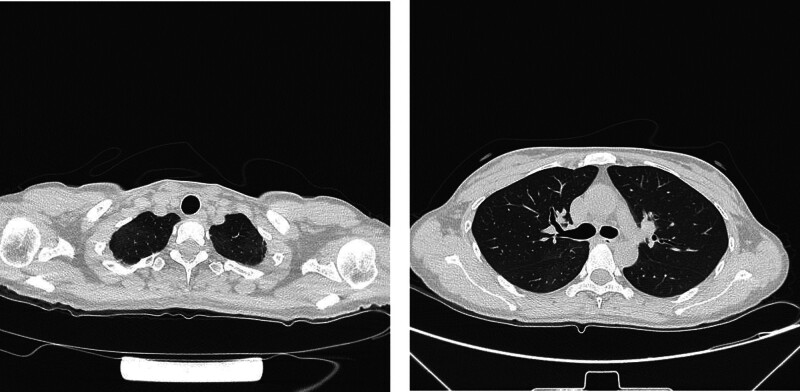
CT scan showing that the lung lesions were completely absorbed after 10 d of glucocorticoid treatment.
